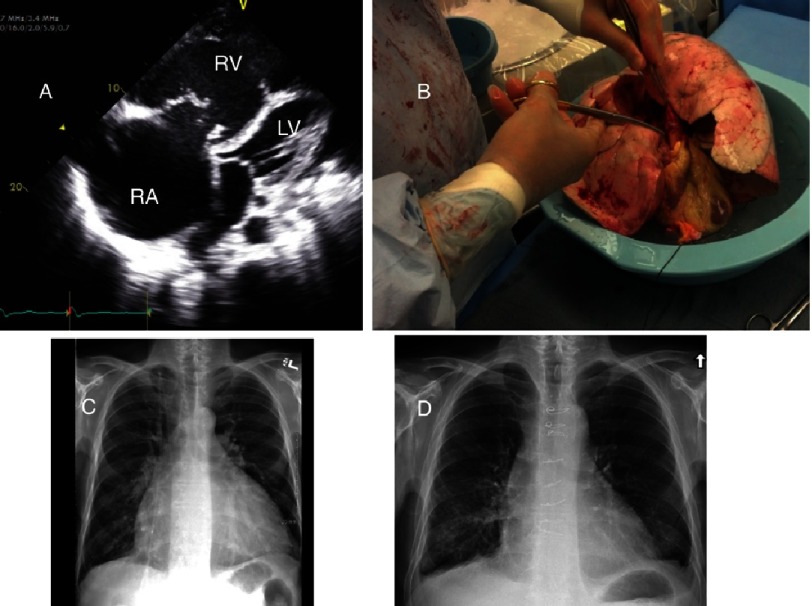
55 year-old male with Idiopathic Pulmonary Arterial Hypertension and severe refractory right heart failure despite therapy with intravenous epoprostenol, requiring continuous ambulatory dopamine infusion for inotropic support. Apical 4-chamber echocardiographic view (A) demonstrates massive dilatation of the right atrium (RA) and right ventricle (RV) impairing left ventricular (LV) filling. The patient underwent a combined heart-lung transplant (B) and is asymptomatic with normal cardiac and pulmonary function 2 years post-transplant. Chest radiograph pre (C) and post (D) transplant.
